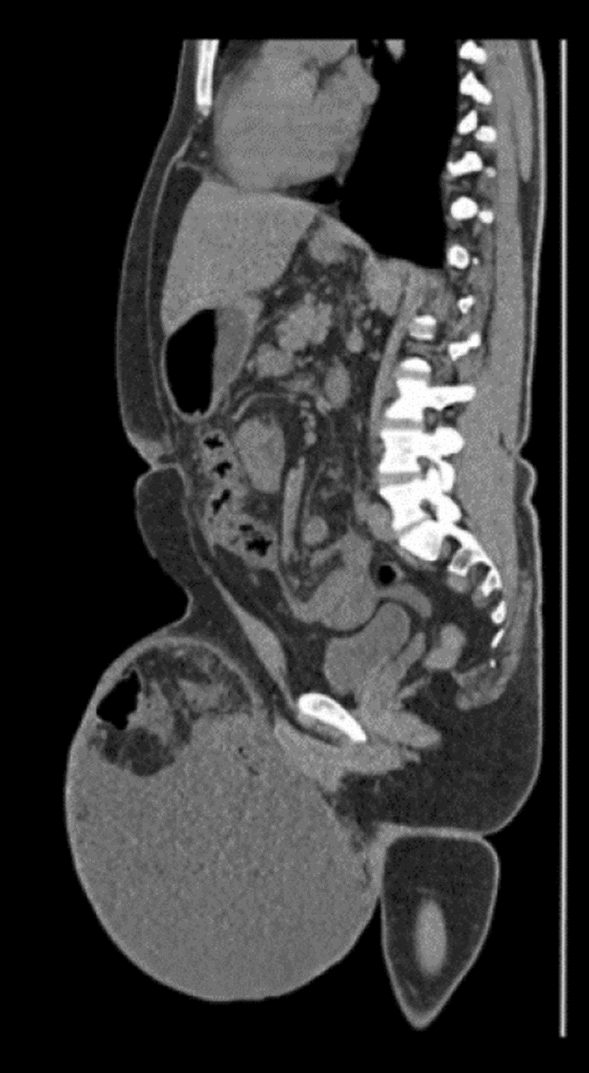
CT scan of the patient’s abdomen before PPP
